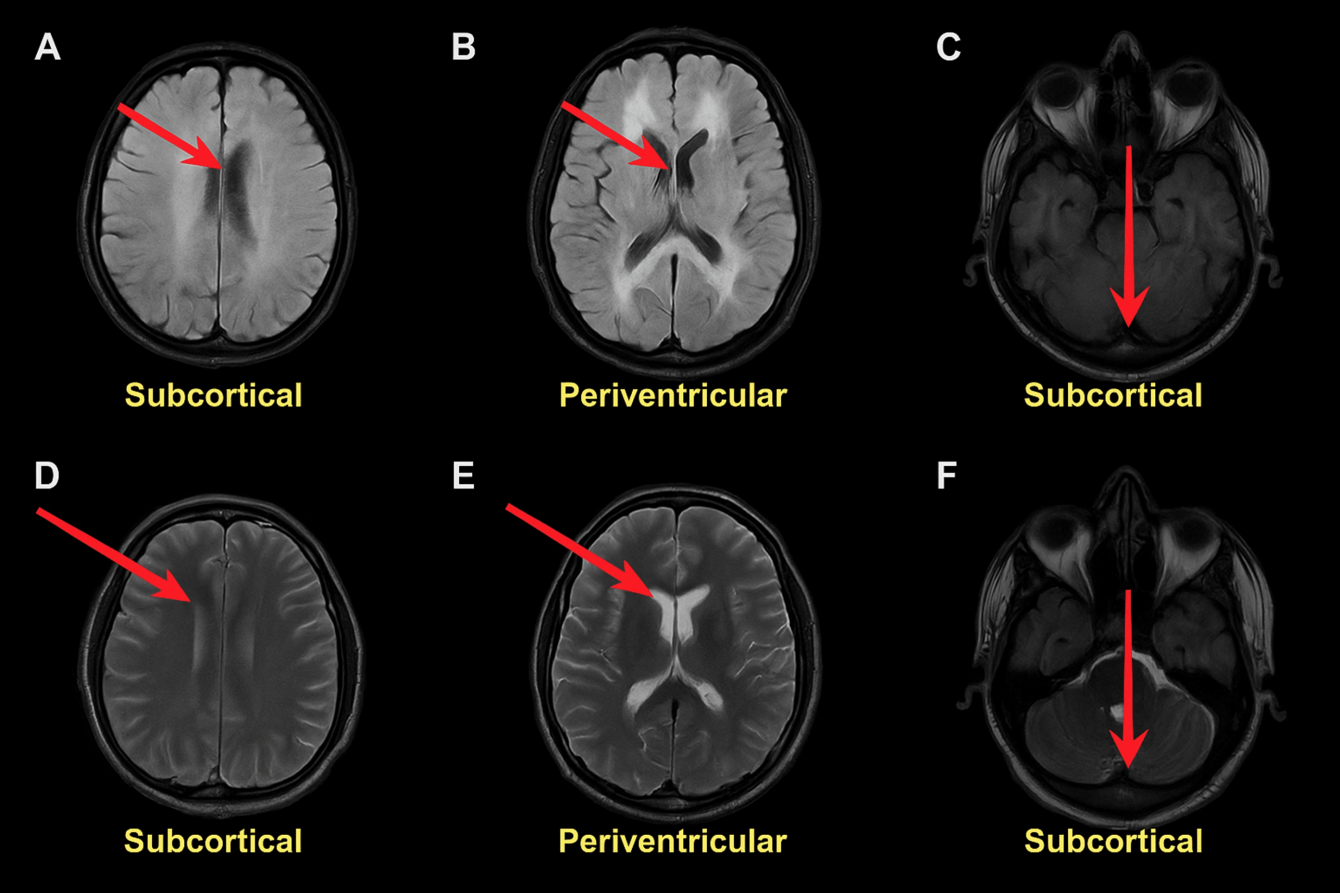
MRI brain Axial brain MRI demonstrating diffuse, symmetric white-matter hyperintensities (red arrows) involving the subcortical (A, C, D, F) and periventricular (B, E) regions on T2/FLAIR and T2-weighted sequences. The deep gray nuclei, brainstem, and cerebellar nuclei are spared. These imaging findings are typical of toxic leukoencephalopathy secondary to heroin vapor inhalation (“chasing the dragon”). T2/FLAIR: T2-weighted fluid-attenuated inversion recovery
